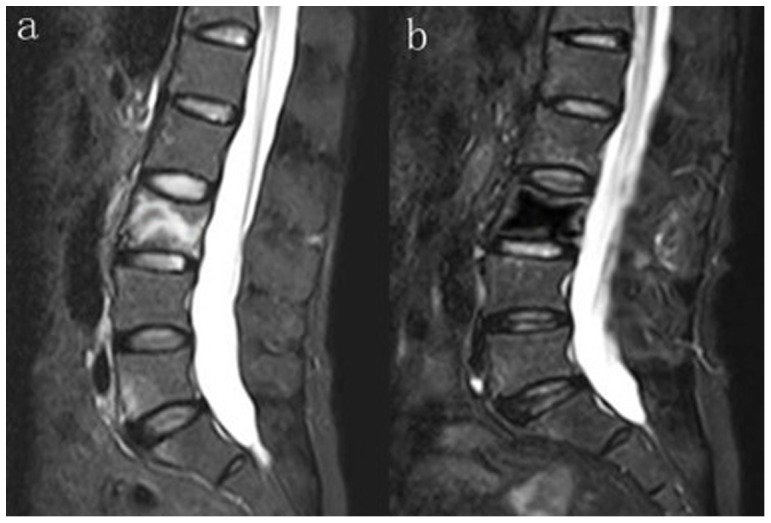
T2-weighted image of MRI in the sagittal plane. (a) Preoperative photograph of VCF of L3 (b) Postoperative photograph of Grade II of degenerative disc above the treated vertebral.
